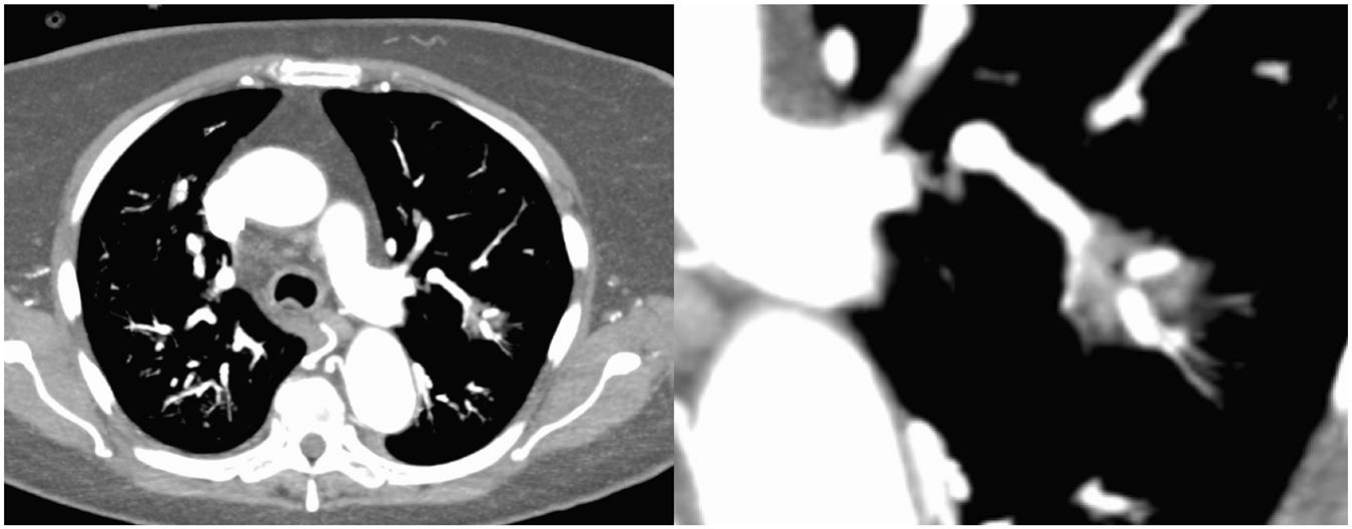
Overview (left) and close-up (right) computed tomography pulmonary angiography images of a 53-year-old immunocompromised patient due to kidney transplantation. The images demonstrate the vessel interruption within the sub-solid lesion.
